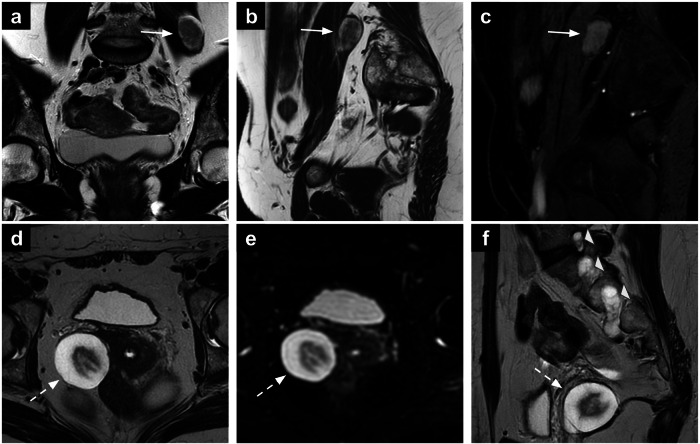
Incidental finding of intramuscular schwannoma in a patient with suspected endometriosis (a–c). A well-circumscribed mass (arrow) showing intermediate-to-high SI on T2-WI is seen in the left psoas muscle (a, b). On post-contrast T1-WI, the lesion shows homogeneous enhancement (c). Cystic degeneration of a schwannoma in a 44-year-old patient (d–f). The lesion (d–f) demonstrates high SI on T2-WI (d) and low *b*-value DWI (e). On sagittal T2-WI (f), there is evidence for multiple lesions with similar signal characteristics associated with the exiting sacral nerve roots (arrowheads). WI, weighted images
